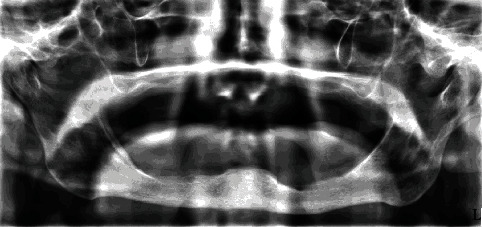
Orthopantomographic image showing no underlying bone resorption or defect.
